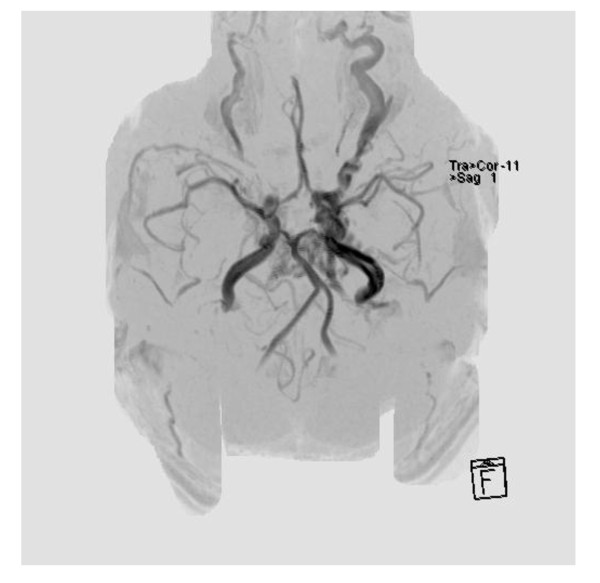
MRI of the brain with contrast. Increased flow in the left cavernous sinus and engorgement of the left ophthalmic vein.
